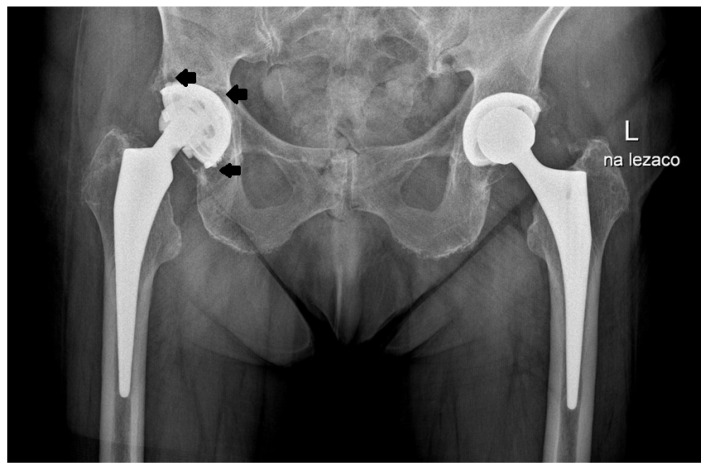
X-ray images of a patient who qualified for right hip revision surgery. The arrows indicate the loosening of the acetabular element.
